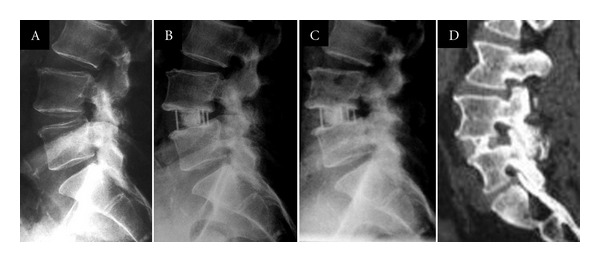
Case example #1. (a) Preoperative X-ray (b) 3-month X-ray (c) 12-month X-ray (d) 12-month computed tomography sagittal reconstruction.
